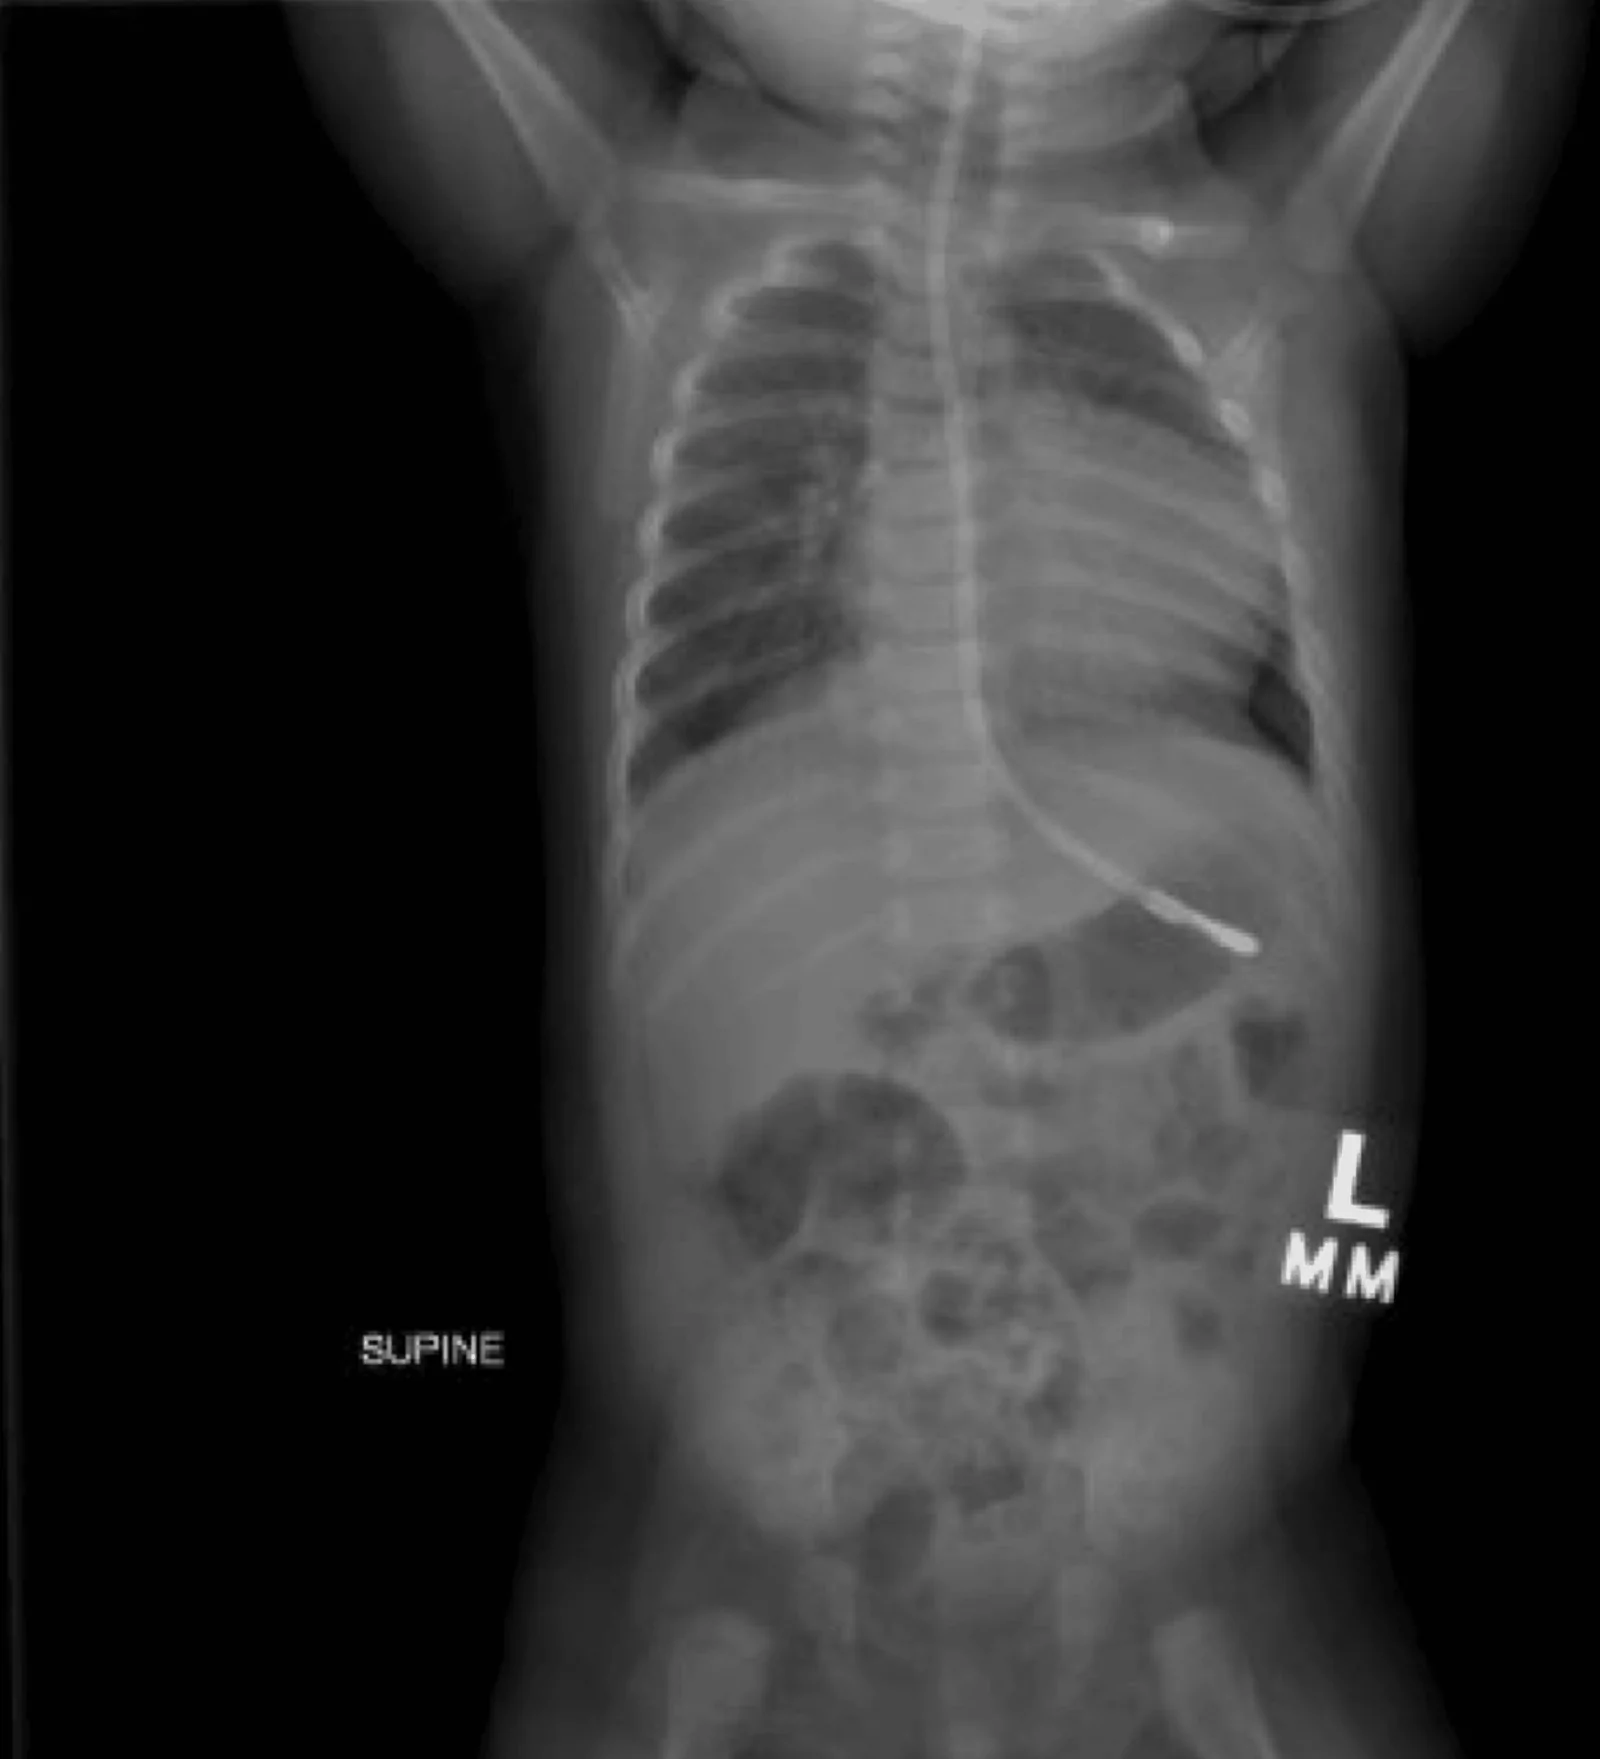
Chest X-ray at three months showing cardiomegaly The heart is enlarged but is not significantly changed compared to the X-ray at one month.
